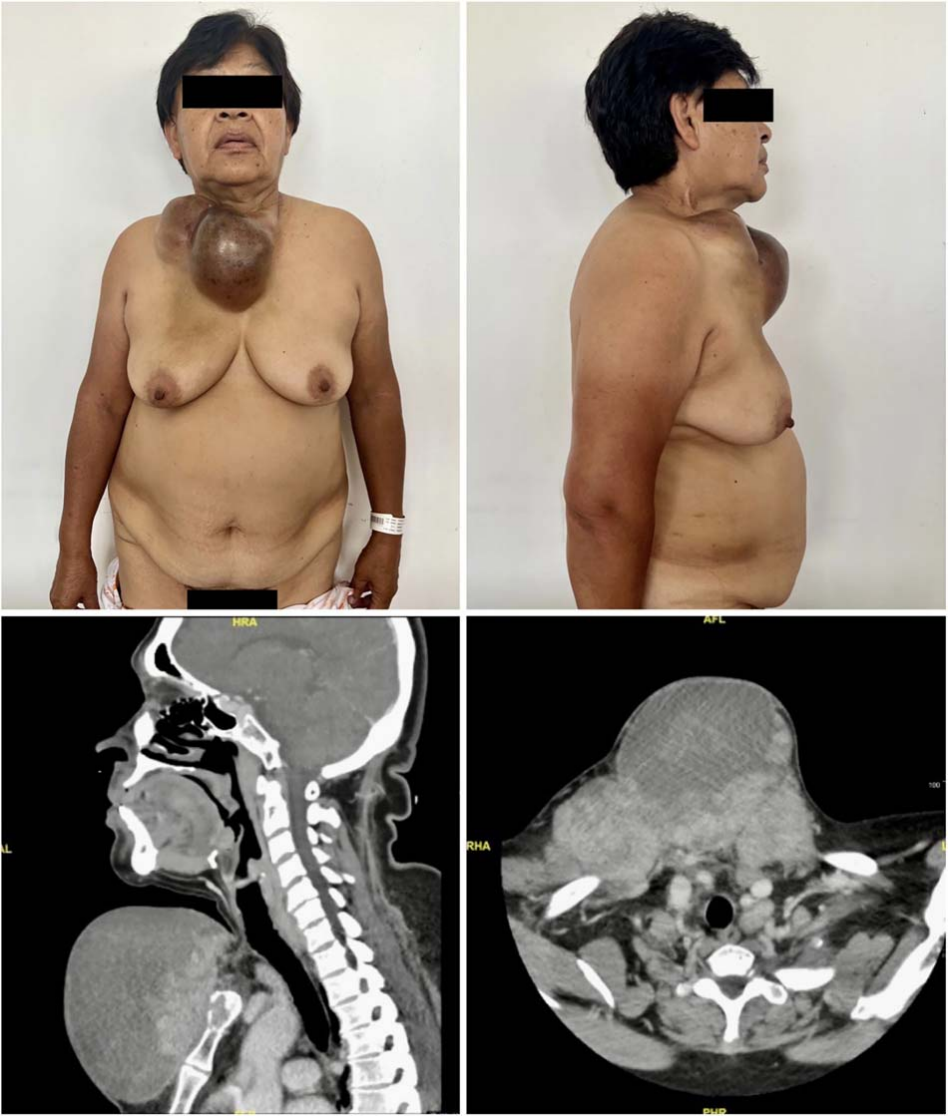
Preoperative photographs (upper, left and right) showing a large, hardened, bilobulated mass measuring 14 × 21 cm across the supraclavicular, infraclavicular, and presternal regions. The CT scans (lower, left and right) showing the posterior surface of the mass in contact with the pectoralis major muscle in the midline, the cortex of the sternal manubrium and the medial ends of both clavicles, and a poor interphase with the left sternocleidomastoid muscle at its caudal insertion.
